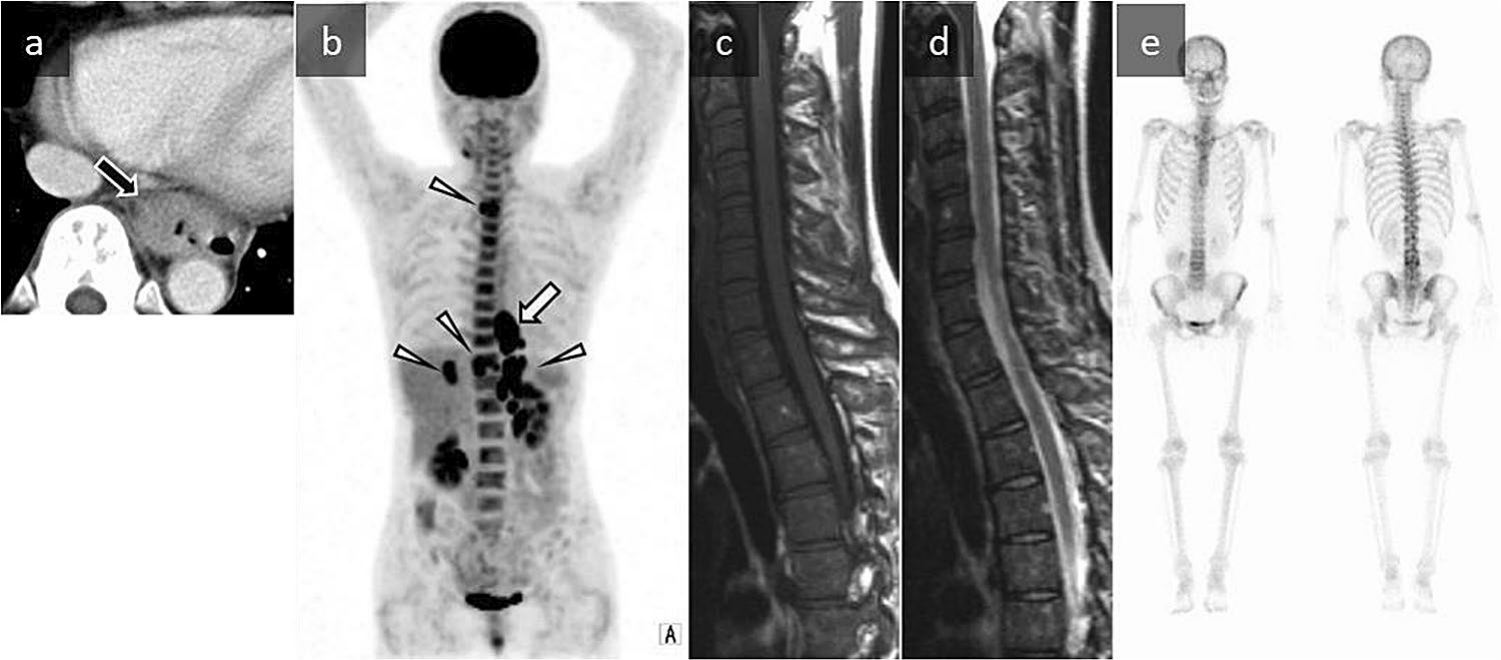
A 52-year-old woman with G-CSF-producing carcinoma of the esophagus. a Axial contrast-enhanced CT shows a well-defined and nonnecrotic mass 4.6 cm in diameter located in the esophagus (black arrow). b An ^18^F- FDG-PET/CT maximum intensity projection image demonstrates marked FDG uptake in the esophageal tumor (maximum standardized uptake value = 32.4, white arrow), lymph nodes, and liver metastases (white arrowheads) with diffuse uptake in the bone marrow of the spine. The splenic uptake is slightly more intense than the hepatic uptake. c, d Spinal sagittal MRI shows that the signal intensity of the bone marrow is lower than that of an age-matched healthy woman on spin echo T1- and turbo spin echo T2-weighted imaging. e Bone scintigraphy shows no significant uptake in the spine
